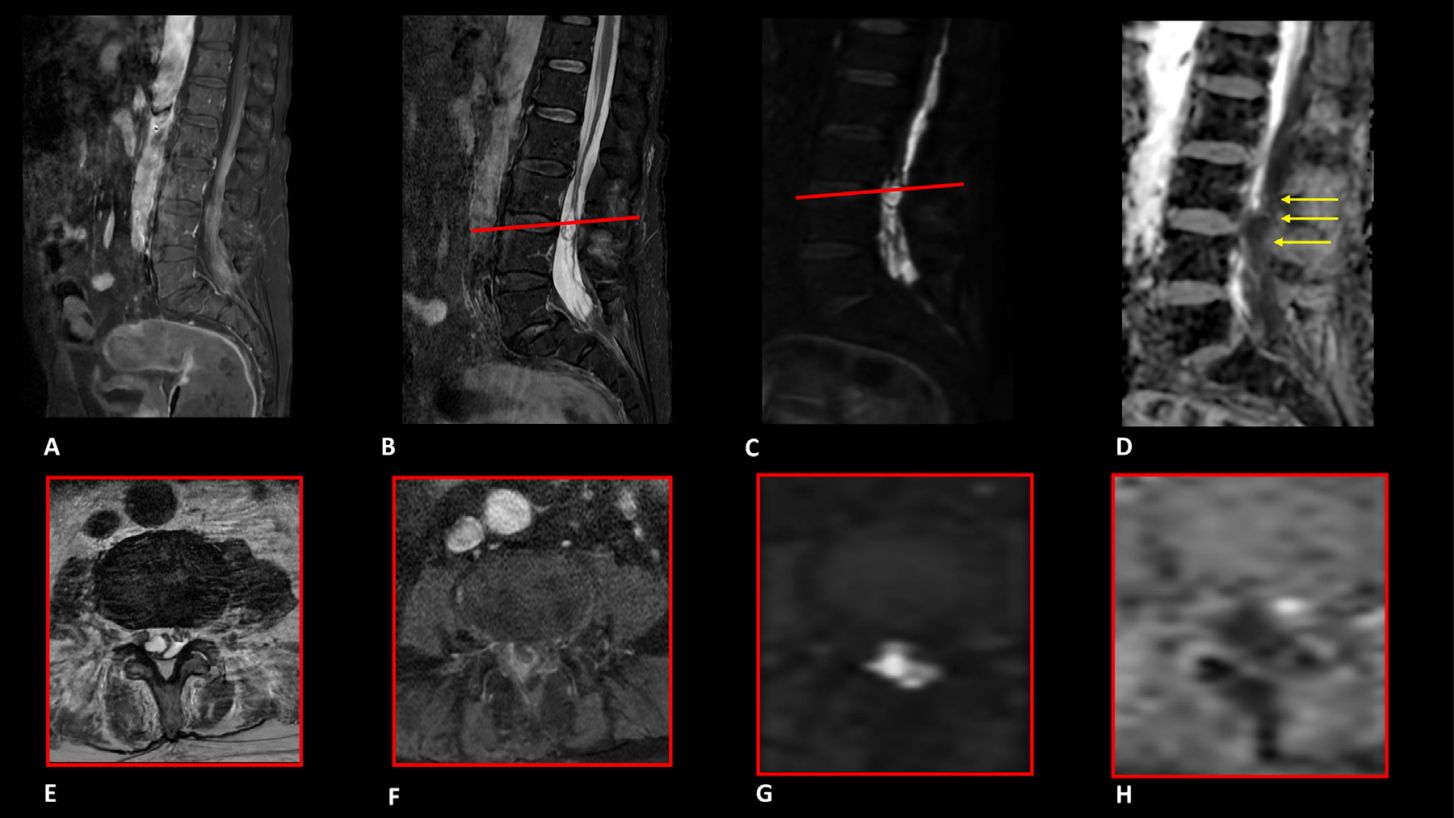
Magnetic resonance imaging of the lumbar spine. Postcontrast sagittal (A) and axial (F) T1 weighted image showing mild post-contrastographic enhancement of a suspected intradural collection in the lumbosacral region (L3-L4 level); Sagittal (C) and axial (G) DWI showed marked restricted diffusion with marked hyposignal on ADC (sagittal D and axial H); in addition, there were signs of arachnoiditis affecting the roots of the cauda which were particularly glued together, in an antigravity position, best appreciated on sagittal STIR (B) and T2 axial image (E).
